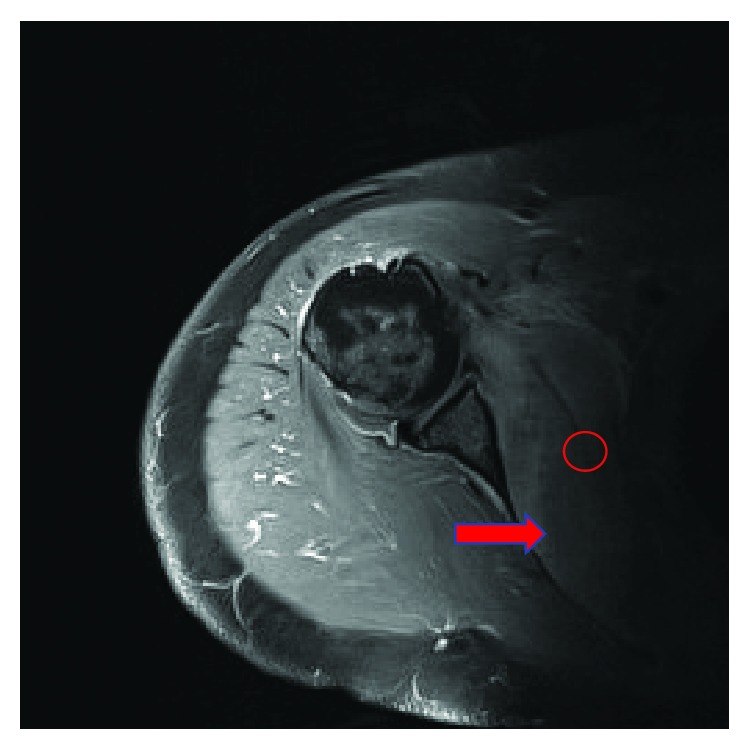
MRI of Rt. shoulder coronal T1 sequence demonstrating odematomous subscapularis muscle.
